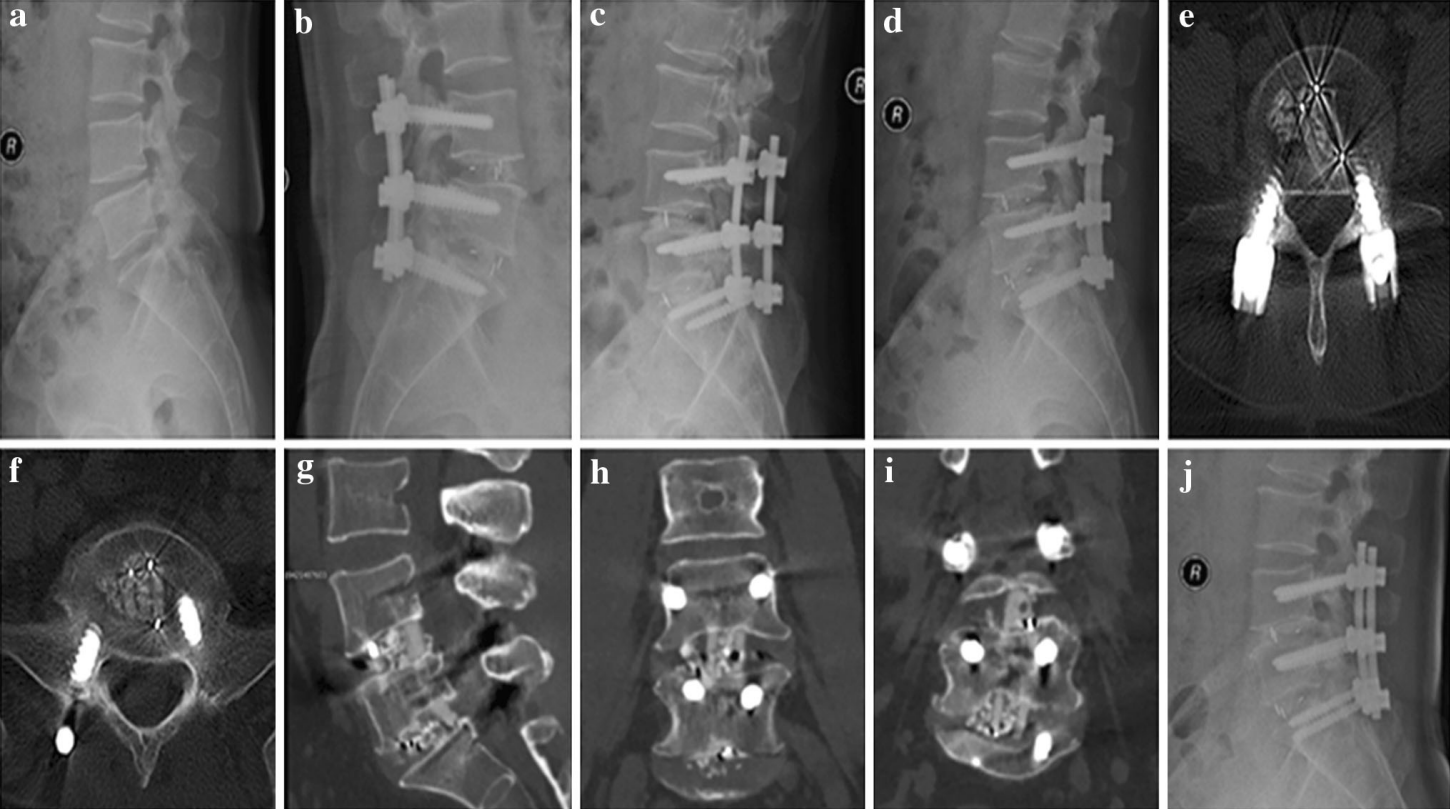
A 58-year-old male who underwent 2-level TLIF with PEEK cages for lumbar reconstruction. The preoperative radiograph (a). The 1-week postoperative and 3-month and 6-month follow-up radiographs (b, c, d). The CT or 3D-CT scan (e, f, g, h, i) shows that the autogenous bone granules fill the cages and achieve bony fusion with adjacent endplates by the 12-month follow-up. A lateral radiograph (j) at the final follow-up shows satisfactory bony fusion and no obvious migration, radiolucent gap or subsidence
